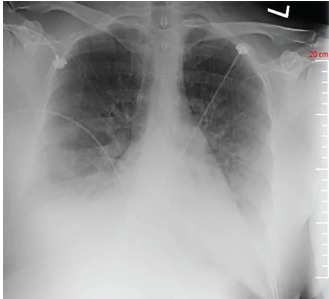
Anterior-posterior chest radiograph demonstrating findings consistent with fluid overload.
